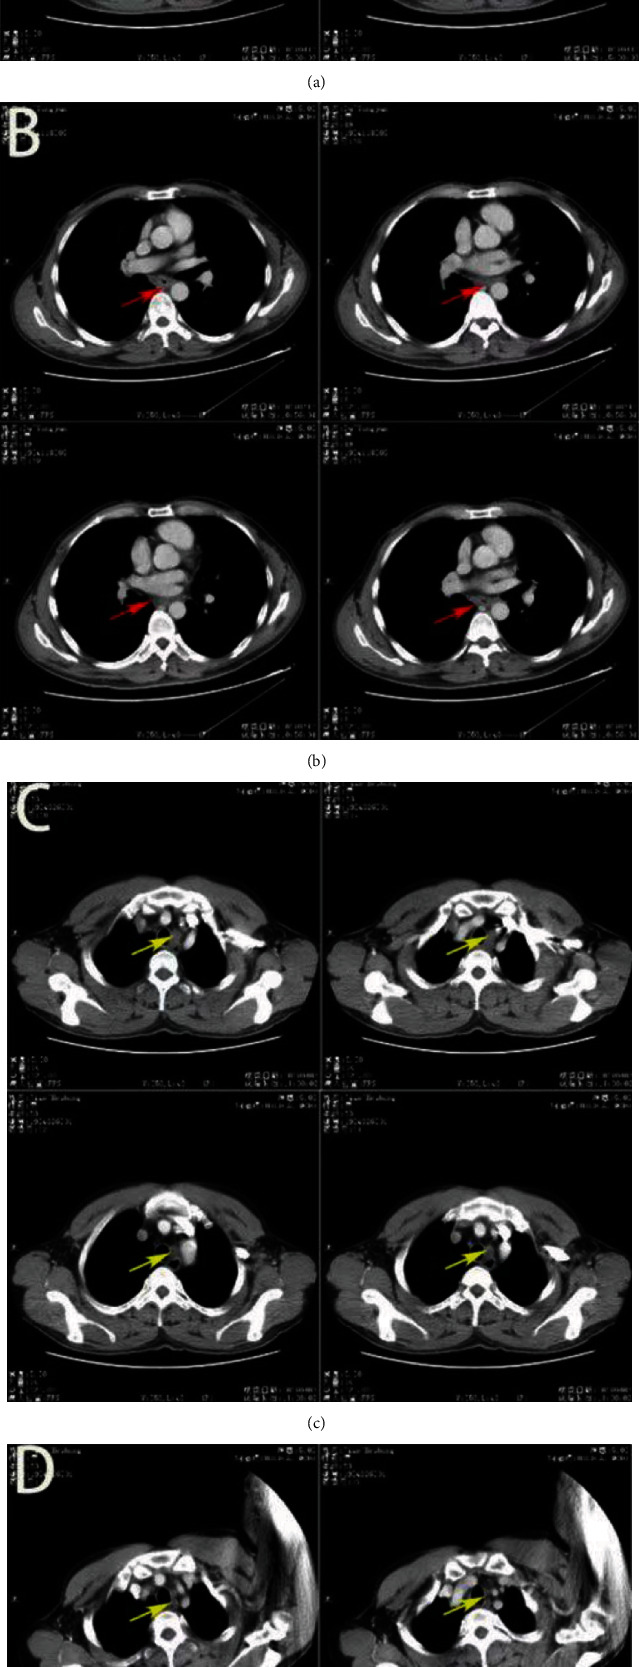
CT images of a case who reached pCR pre-TPA and post-TPA treatment ((a) for esophageal tumor of pre-TPA, (b) for esophageal tumor of post-TPA, (c) for positive lymph node of pre-TPA, and (d) for positive lymph node of post-TPA).
